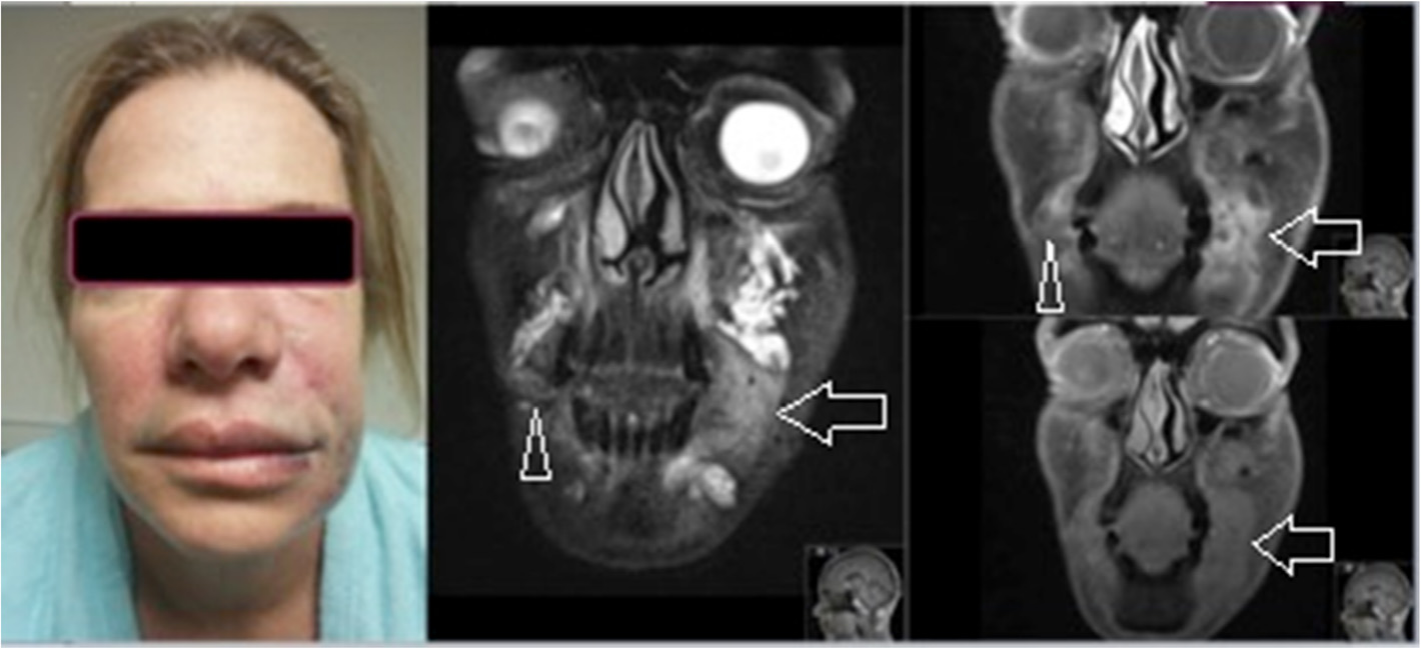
Acute allergic reaction to hyaluronic acid in the left nasolabial sulcus and mouth angle sulcus region (*open arrows*) on coronal MRI (T2FS, FS T1FS with gadolinium). This soft tissue reaction is often reffered to “cellulitis-like.” Note the normal filler in the right lower infraorbital triangle and nasolabial sulcus (*arrowhead*)
